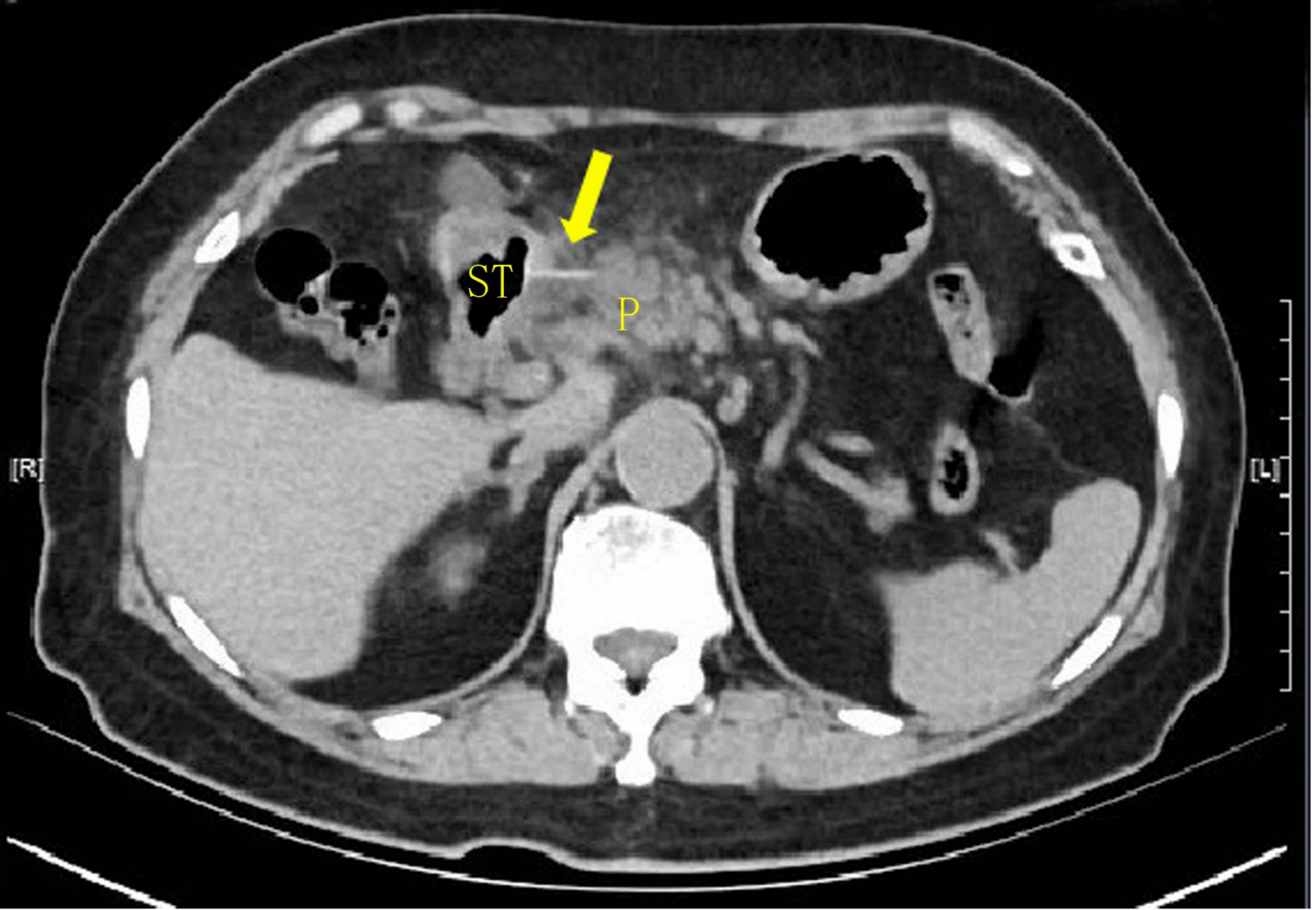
Upper abdominal CT image on admission showing the fishbone (arrow) penetrating the gastric antrum and piercing the head of the pancreas P: pancreas, ST: stomach, CT: computed tomography
